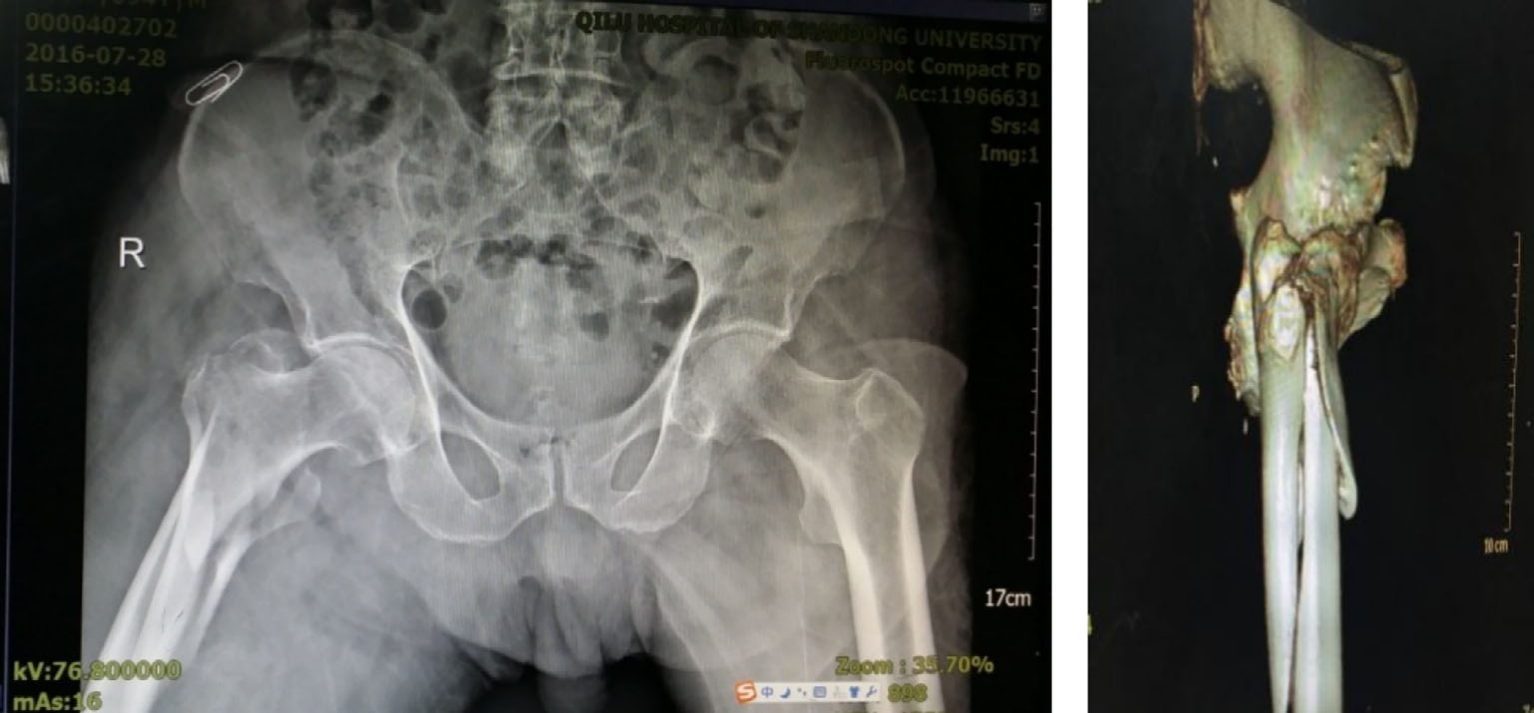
Preoperative X‐ray and CT.
